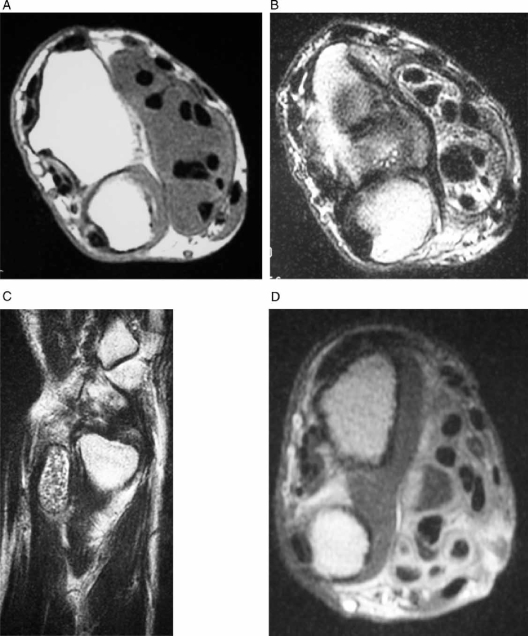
A: Axial T1-weighted MR image of the right distal forearm demonstrates a mass with low signal intensity surrounding the flexor tendons. B: Axial T2-weighted MR image showed a mass with high signal intensity mass and many rice bodies with low signal intensity. C: Sagittal T2-weighted MR image of the right distal forearm showed enlarged tendon sheaths and many small bodies with low signal intensity. D: Axial T1-weighted MR images of the right distal forearm after the administration of gadolinium. The mass which surrounded the flexor tendons was moderately enhanced.
